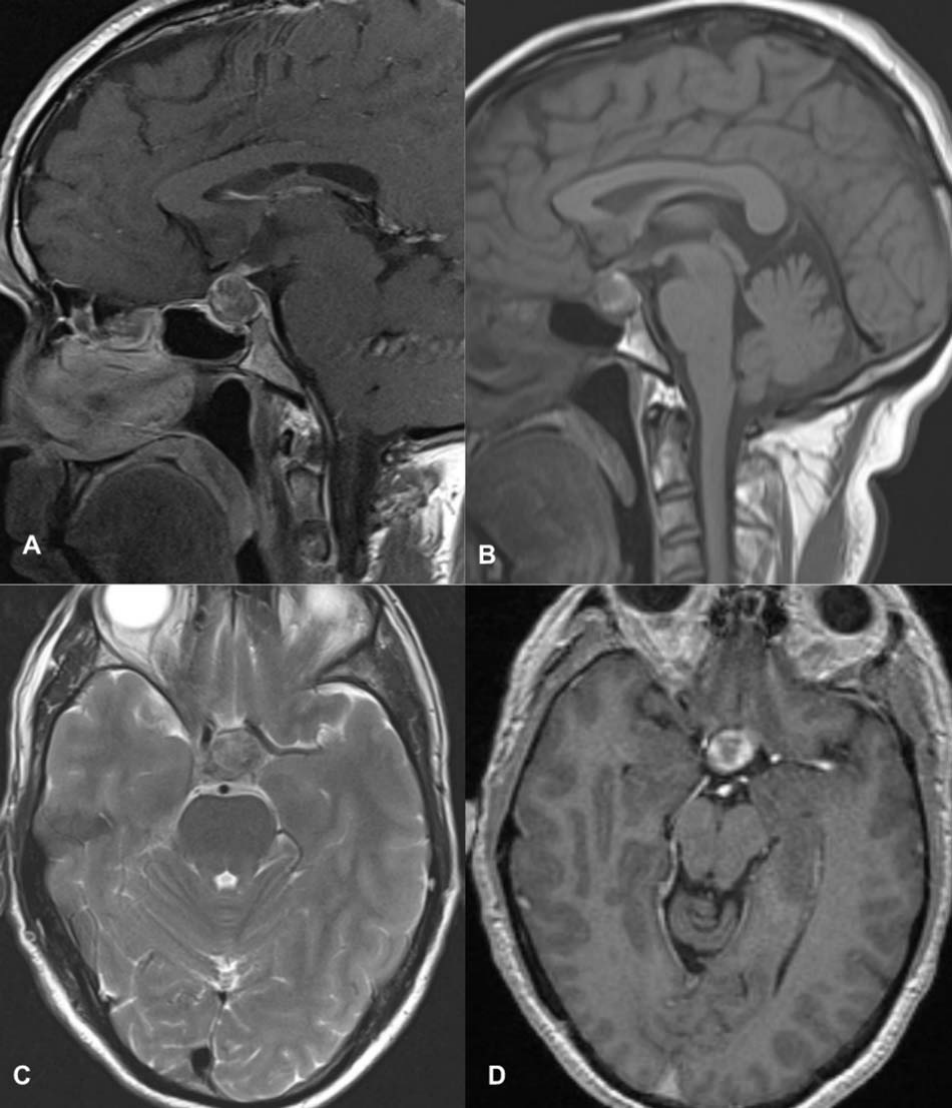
MRI images of post-contrast sagittal T1 (A), sagittal T1 (B), axial T2 (C), and post-contrast axial T1-weighted images (D) showing a 2 cm × 1.6 cm × 1.6 cm sized sellar lesion arising from the pituitary fossa extending superiorly in the suprasellar region and laterally causing a mass effect on the left cavernous sinus with intrinsic high T1 signal in the periphery and signal void on T2-weighted images suggestive of hemorrhage.
